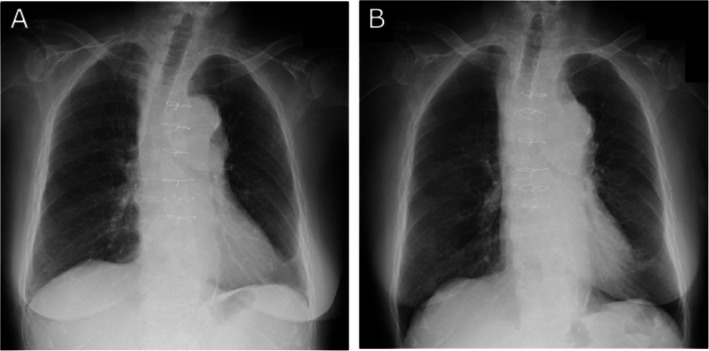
X-ray figure taken in upright position on the day Z−20 (A) and the day just before the first surgery (B)
